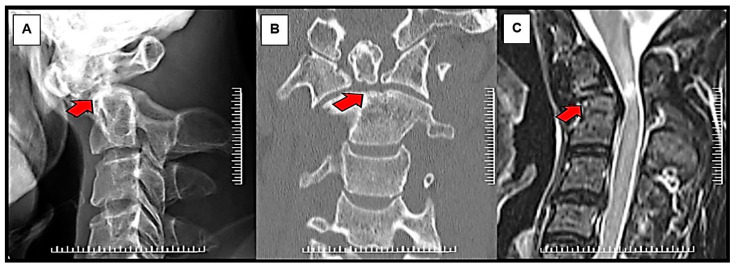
Radiographic images of nonunited type II odontoid fracture with atlantoaxial instability. Atlantoaxial instability with cervical myelopathy caused by nonunited type II odontoid fracture (Red arrows): (A) lateral plain radiograph; (B) coronal CT image; (C) sagittal MR image.
